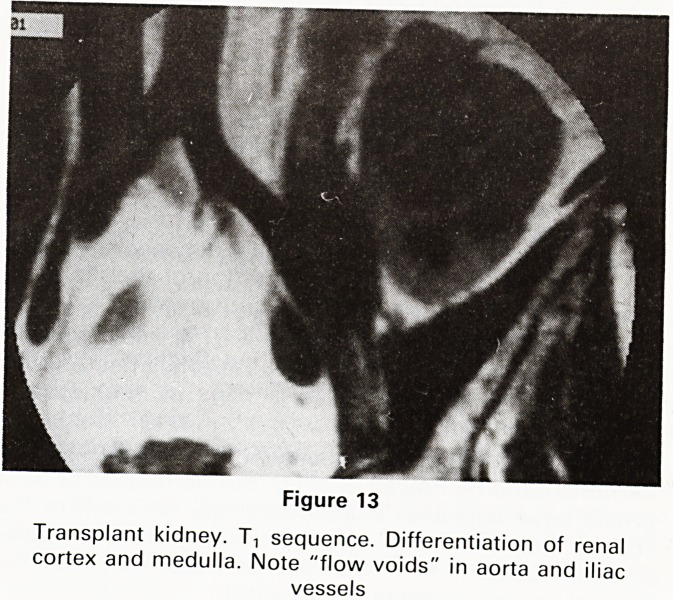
# MRI and the District General Hospital
